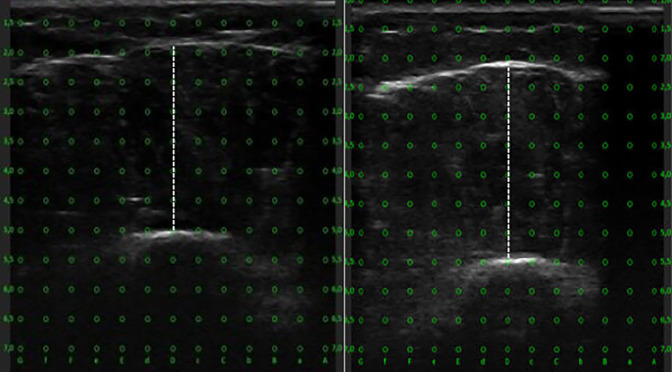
Representative ultrasound images of the lateral deltoid muscle measuring muscle thickness. The left panel shows the proximal region, and the right panel shows the distal region.
